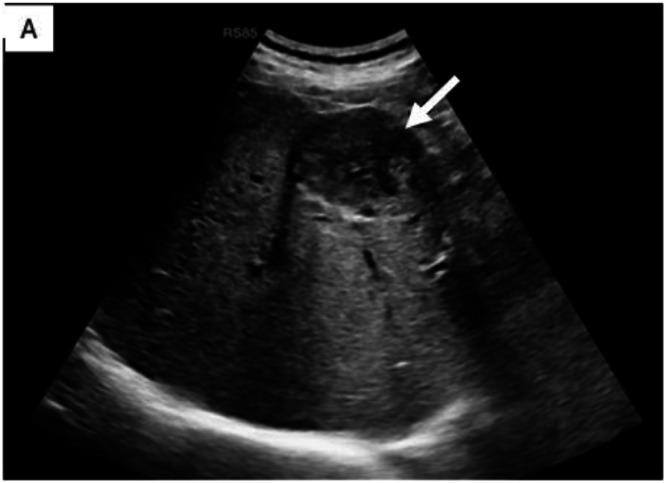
Ultrasound image shows a solid-cystic echoic lesion with posterior acoustic enhancement in cholecystectomy bed (white arrow) (A).
